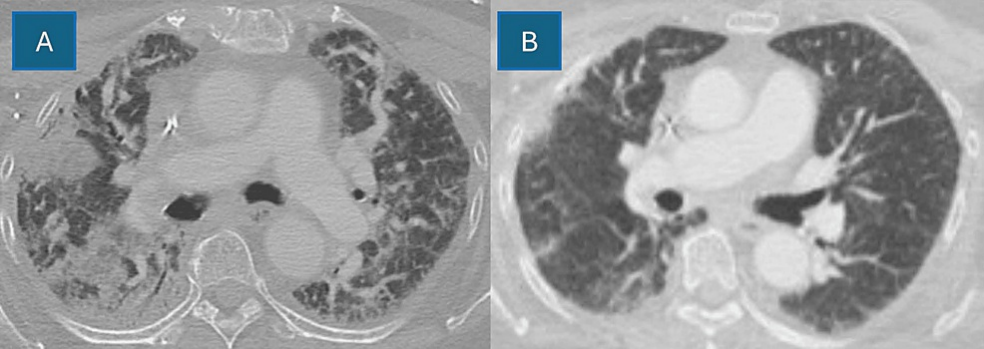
(A) Admission contrasted chest CT demonstrated multifocal airspace disease throughout lung fields, worse on the right. Background subpleural reticulation with underlying interstitial lung disease/fibrosis and diffuse peri-bronchial wall thickening is also present. (B) Comparator chest CT from 2022 with baseline subpleural reticulation and underlying fibrosis
